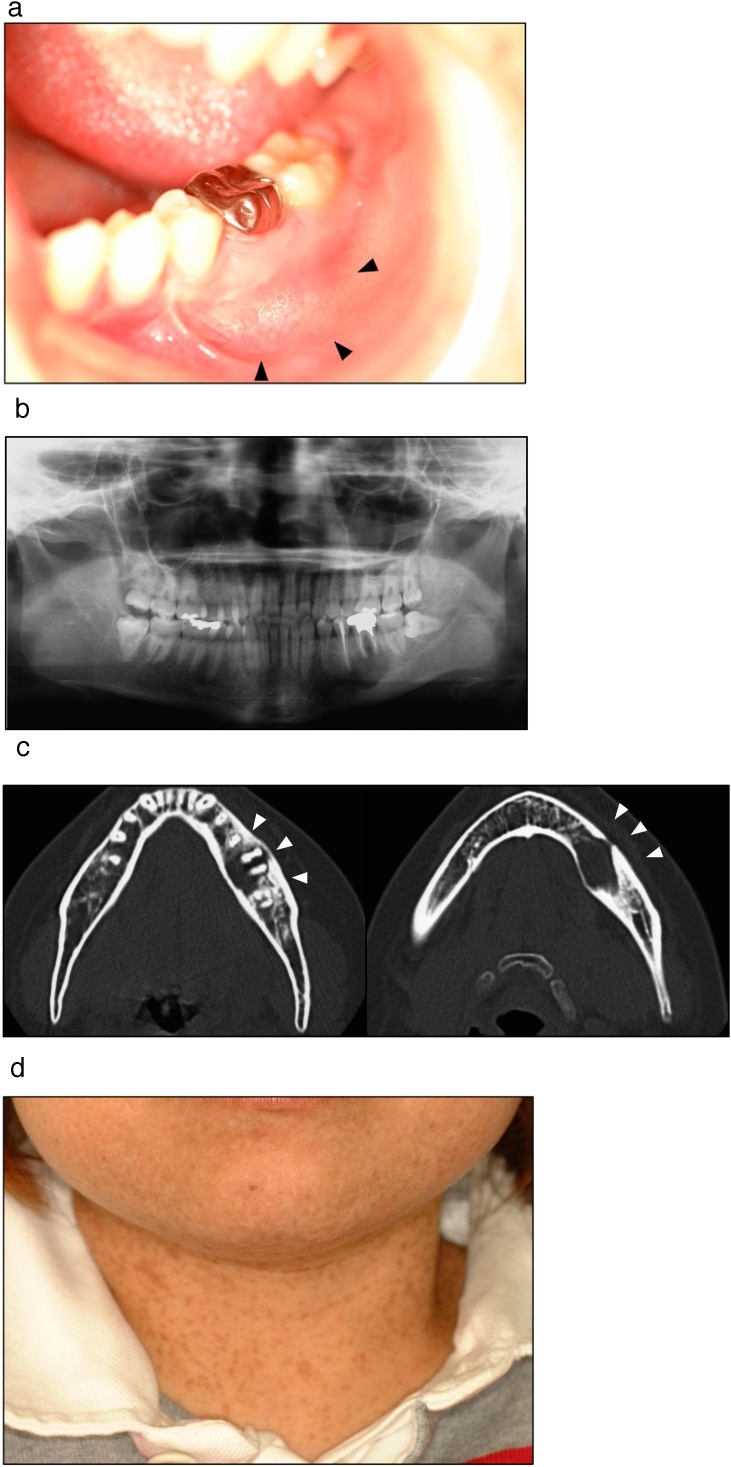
a) Intraoral findings; A bulge of the bone-like hardness of approximately 35 × 20 mm was observed at the gingivobuccal fold of the left mandibular molar. b) Panoramic radiograph showing a well-defined mass in the left mandibular molar region. c) A 14 × 21-mm region was observed in the left mandible molar on CT images. d) Numerous cafe au lait spots on the facial of the skin.
